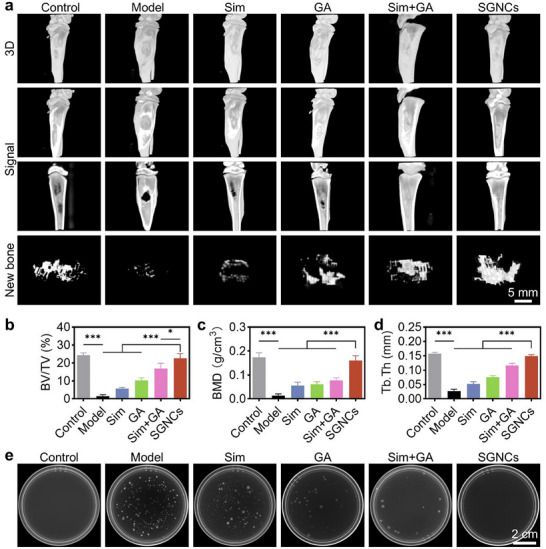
In vivo osteogenic and antibacterial efficacy of SGNCs. (a), Representative micro‑CT 3D reconstruction images of tibial defects. (b–d), Quantitative analysis of BV/TV (b), BMD (c), and Tb.Th (d). e, Bacterial load in bone homogenates assessed by agar plate culture. Data are shown as mean ± SD (n = 3). ^*^
